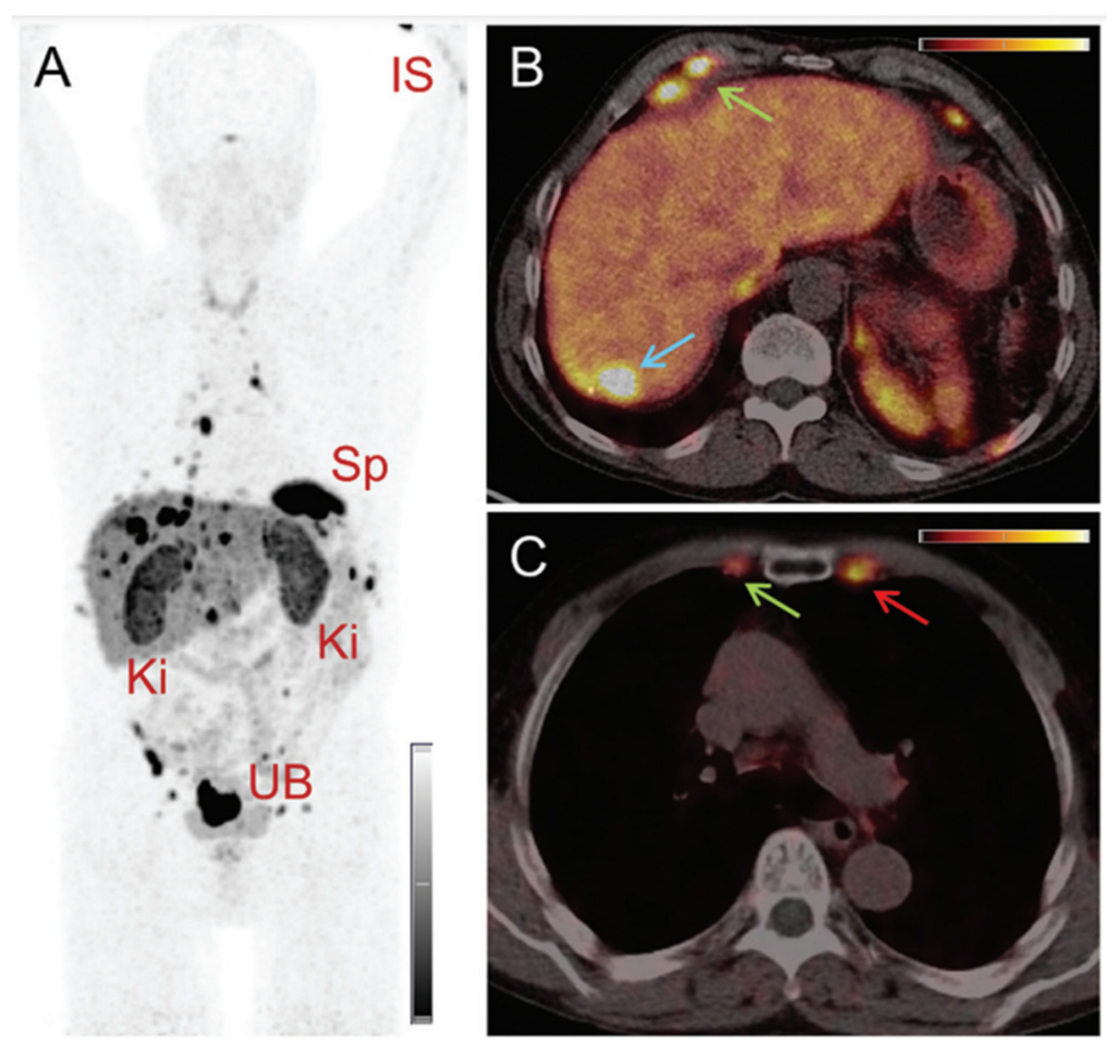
PET/CT images of the same patient acquired 55 min after injection of [ ^68^Ga]Ga-DOTATOC (104 MBq). (IS = site of injection, Ki = kidneys, Sp = spleen, UB = urinary bladder). Reprinted with permission from Baum *et al*. 88, copyright by The Royal Society of Chemistry 2017.
